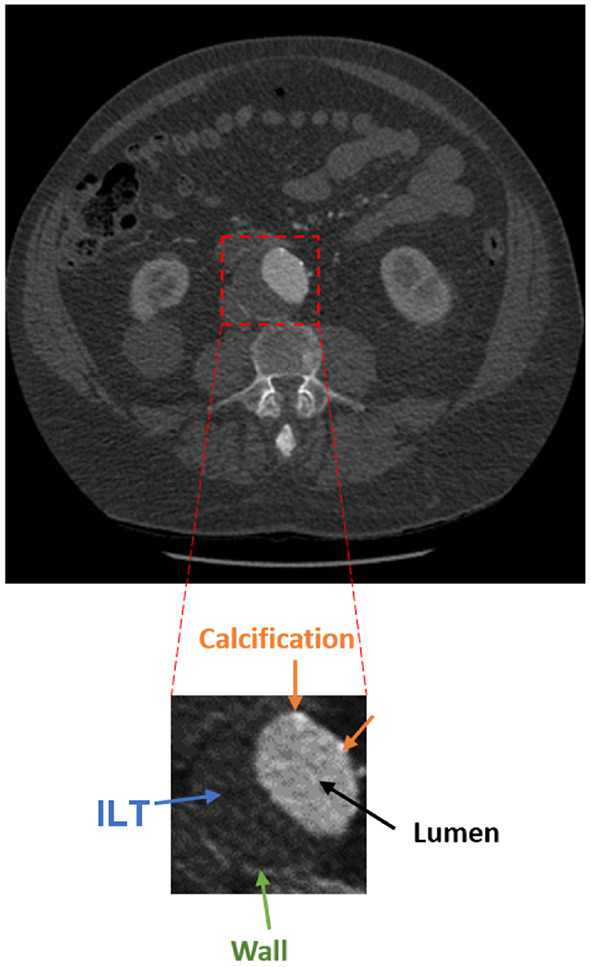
Axial acquisition of an abdominal CT scan showing an AAA. The aorta is a small fraction of the whole image and is composed of the lumen (black), ILT (blue), wall (green), and calcification (orange).
